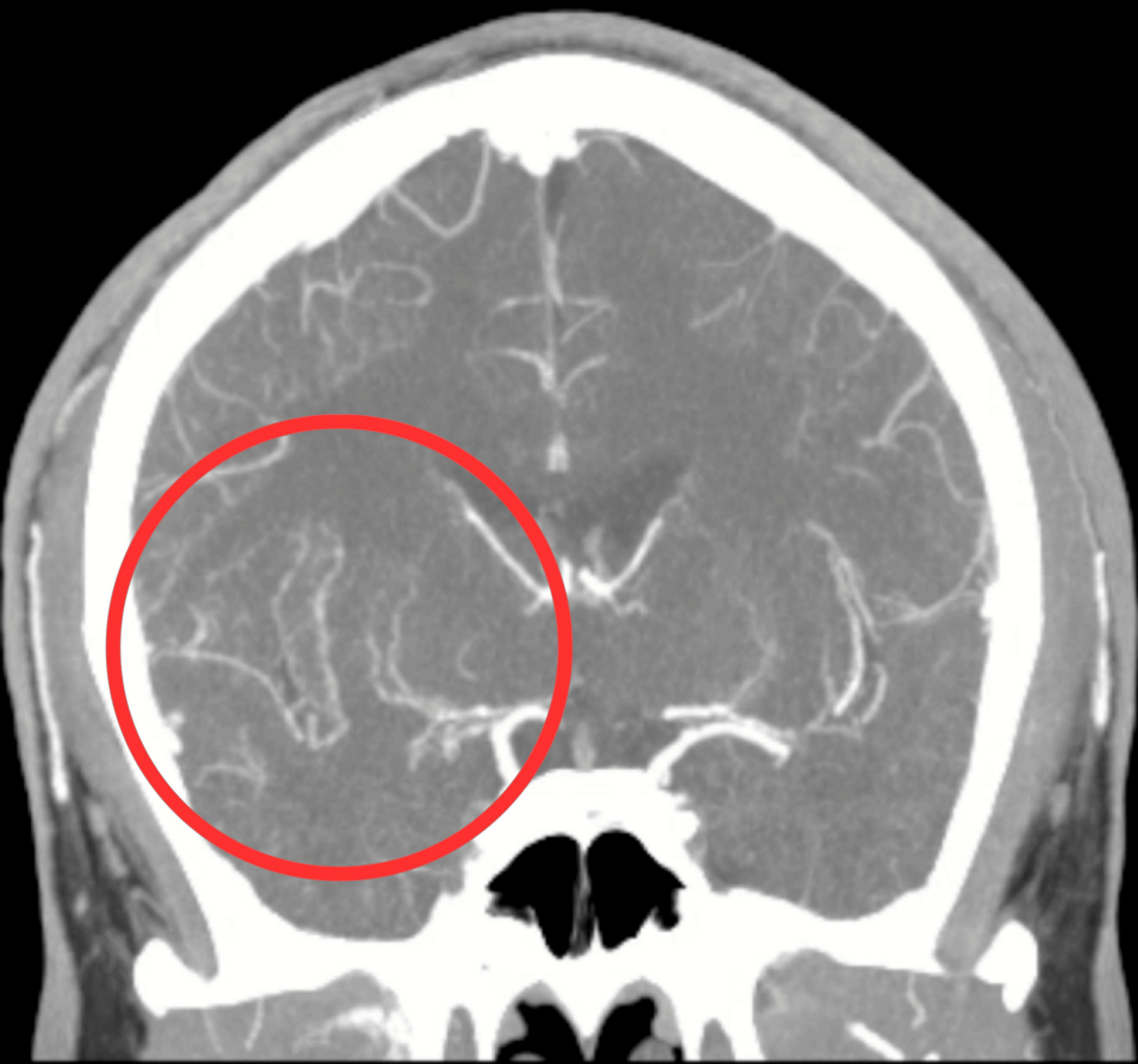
Coronal view of CTA demonstrating the occlusion of the right M1 segment of the middle cerebral artery (red circle), along with extensive collateral vessel formation. CTA: Computed tomography angiography
